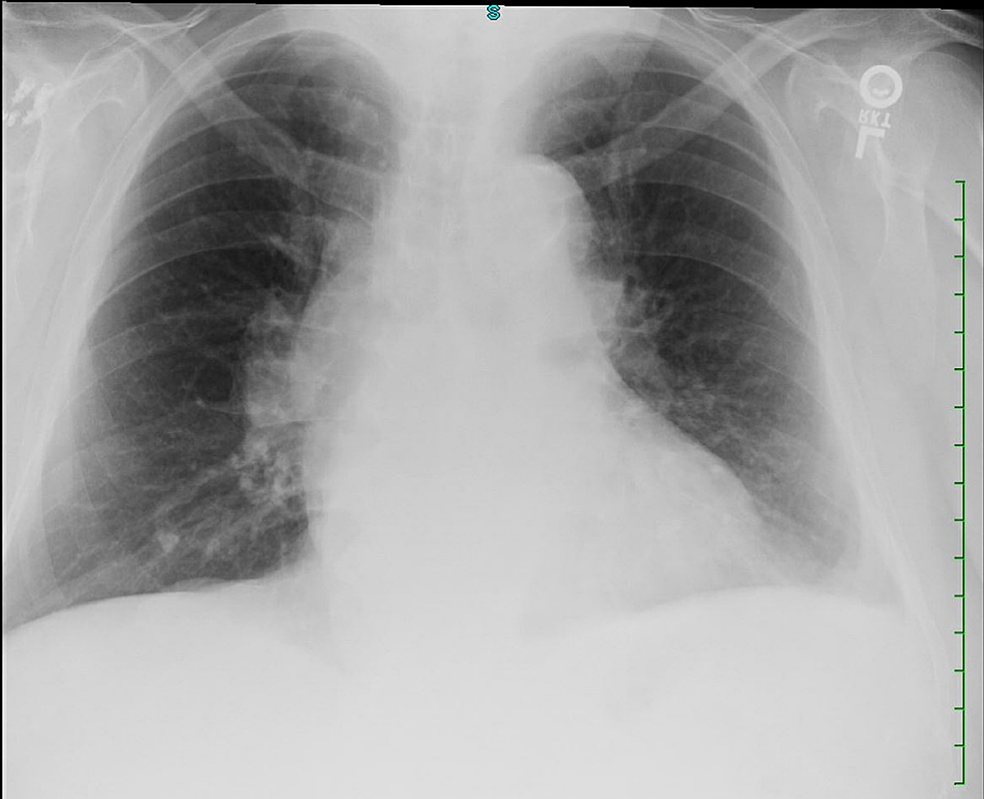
Chest X-ray showing borderline cardiac silhouette with a small left pulmonary effusion but without pulmonary infiltrates or consolidation
